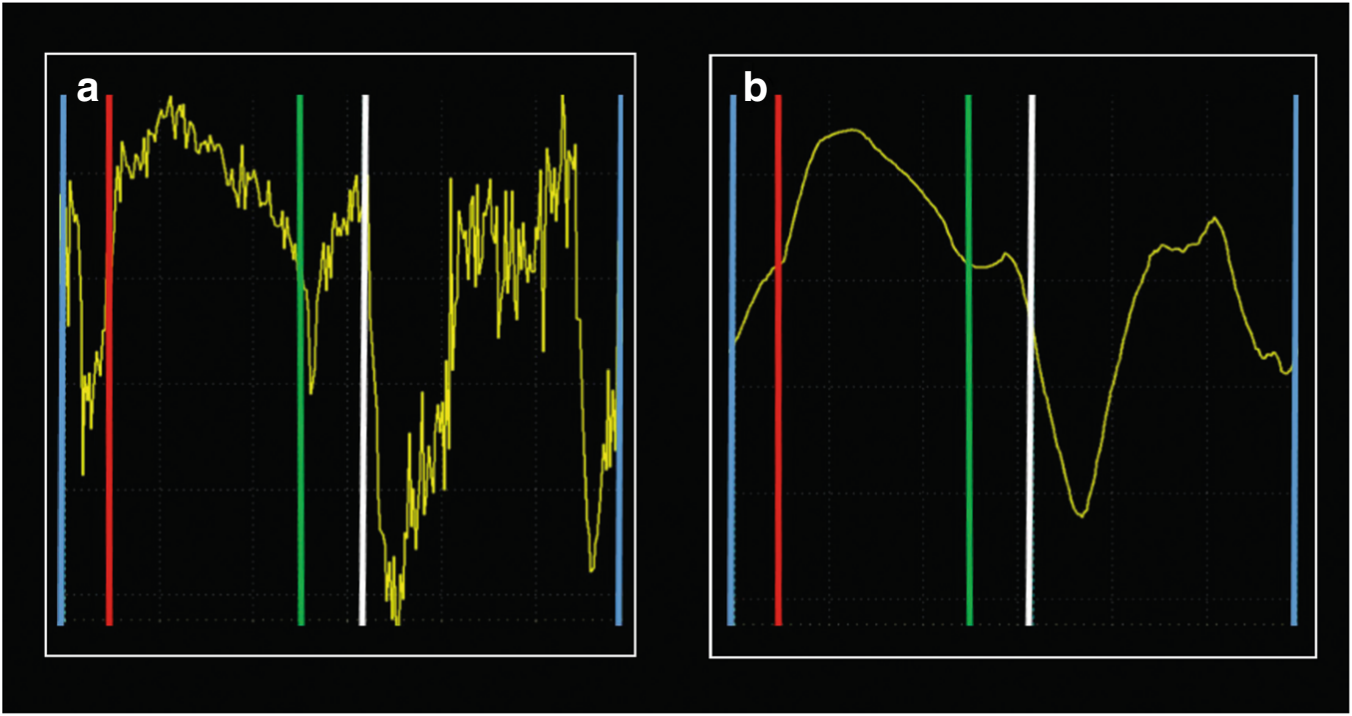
Same loop as in upper panel of Fig. 2, color-coded tissue Doppler velocity curves from a term neonate from the left lateral atrioventricular plane from the apical four-chamber view. The left curve (a) shows the velocities without time smoothing and the right curve (b) shows the velocities with too much time smoothing. Too much and too little time smoothing make interpretation of the curves difficult. Note that it is hard to identify the isovolumic contraction phase, especially in the right panel where the negative slope prior to the ejection phase is missing. Blue vertical lines denote closure of the mitral valve. Red vertical line denotes opening of the aortic valve. Green vertical line denotes closure of the aortic valve. White vertical line denotes opening of the mitral valve
